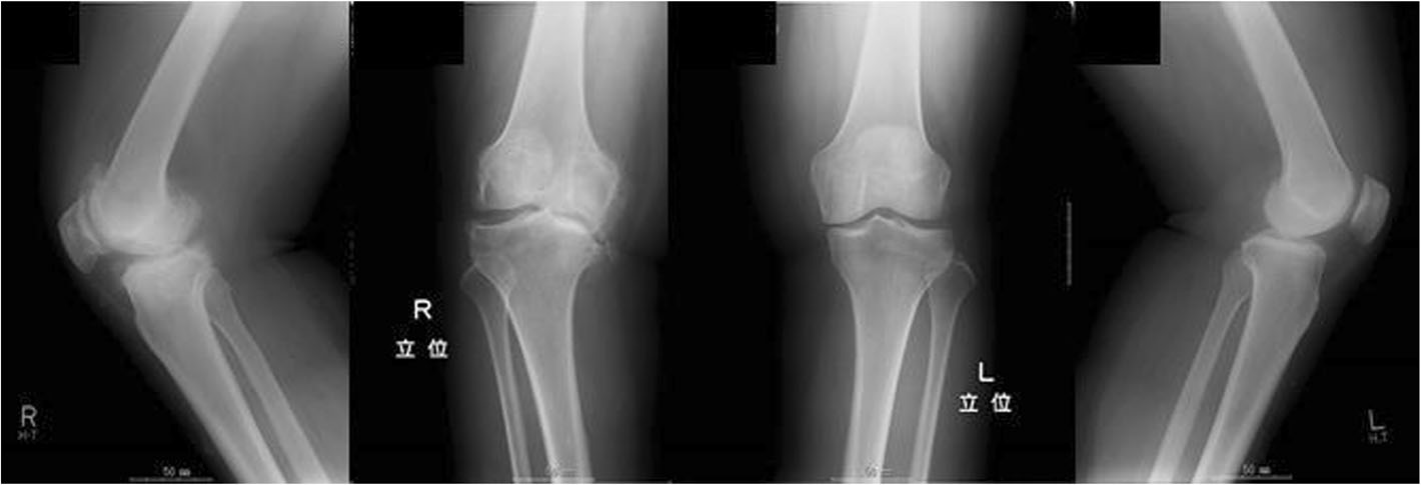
X-ray photograms of the legs
